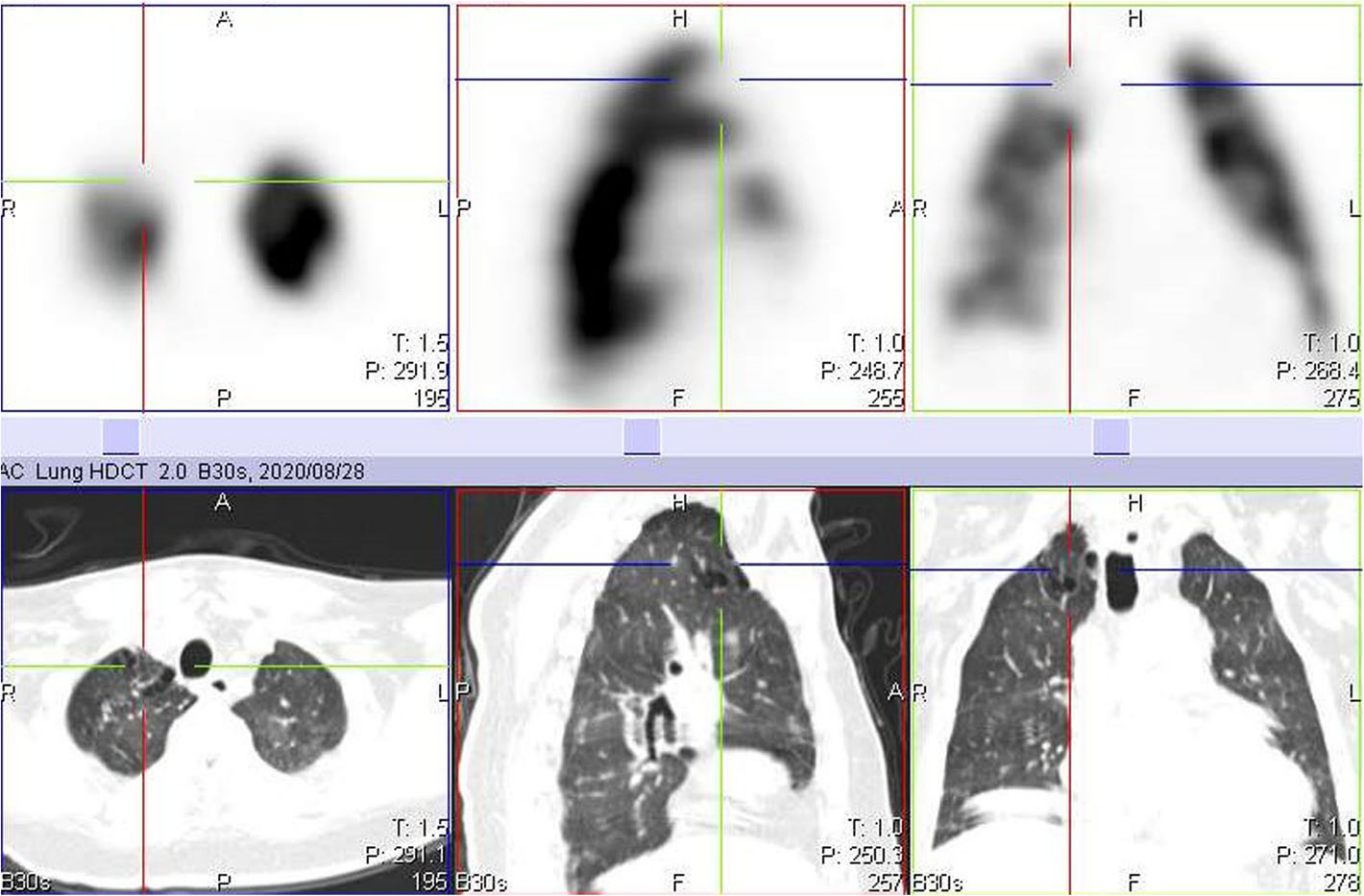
Axial, coronal and sagittal SPECT images of the lungs showing another large perfusion defect in the apical segment of the right upper lobe. However, the corresponding CT images below show structural lung changes with mosaic attenuation in that segment
